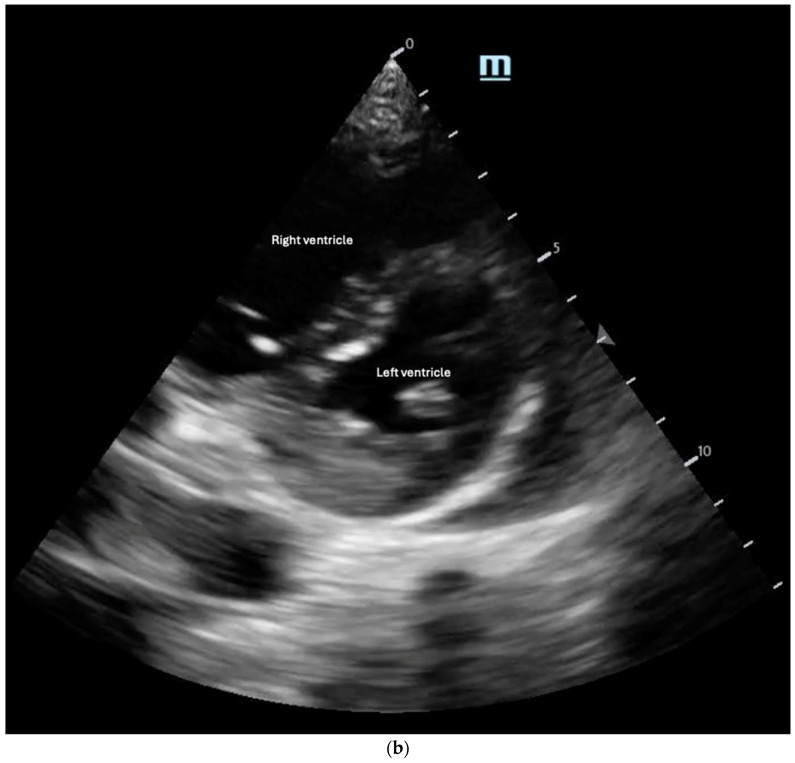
(a) Tricuspid regurgitation velocity (TRV) measured by continuous wave (CW) Doppler in apical 4-chamber view. (b) D sign in parasternal short axis view in diastolic frame. Note flattening of the interventricular septum.
